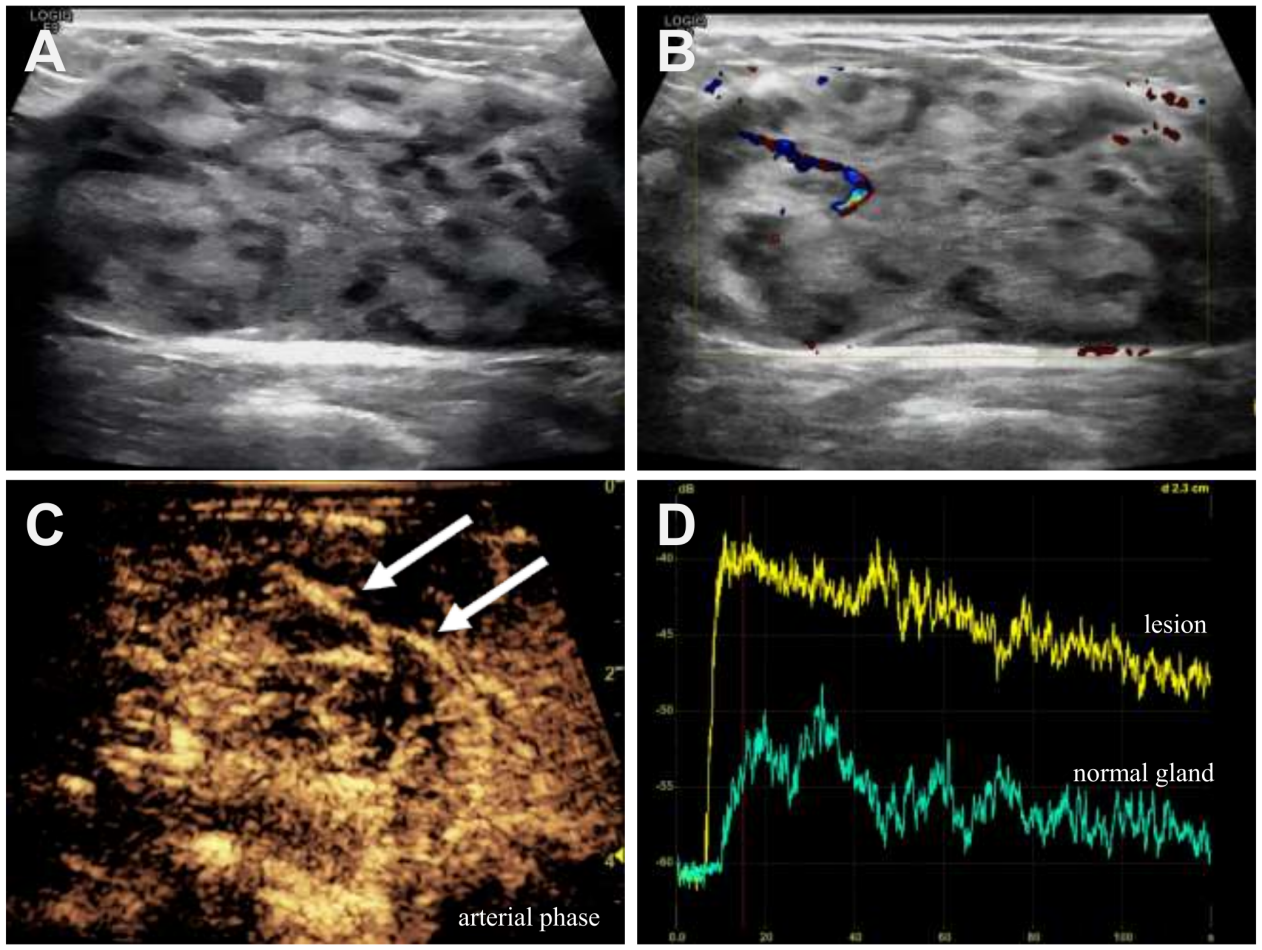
Patient underwent multimodal ultrasound examination for disease diagnosis. (A) Gray - scale ultrasonography showed a 57×51×27mm hypoechoic, thickened, disordered area at 3 o’clock in the left breast. It had ill-defined borders, inhomogeneous internal echo, similar to adjacent glandular tissue. (B) CDFI detected a blood flow signal in the lesion. (C) During the arterial phase, CEUS revealed a “floating vessel” sign (indicated by the arrow) within the lesion, accompanied by homogeneous enhancement of the surrounding tissues. (D) Quantitative CEUS time-intensity curve. The yellow and green curves represent the lesion and the surrounding glandular tissue, respectively.
